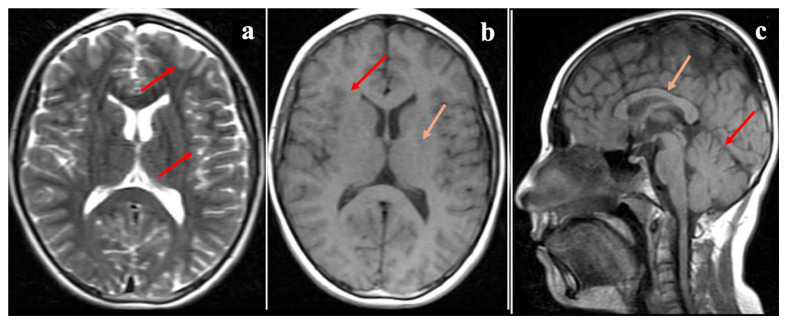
Cranial MR images of the younger sibling (Proband 2, P2) at 12 years of age. (a) T2-weighted axial image demonstrates normal cerebral sulci and gyri (red arrows). (b) T1-weighted axial image demonstrates normal cerebral sulci and gyri (red arrow) and basal ganglia (orange arrow). (c) T2-weighted axial image demonstrates a normal corpus callosum (orange arrow), with no evidence of cerebellar atrophy (red arrow).
